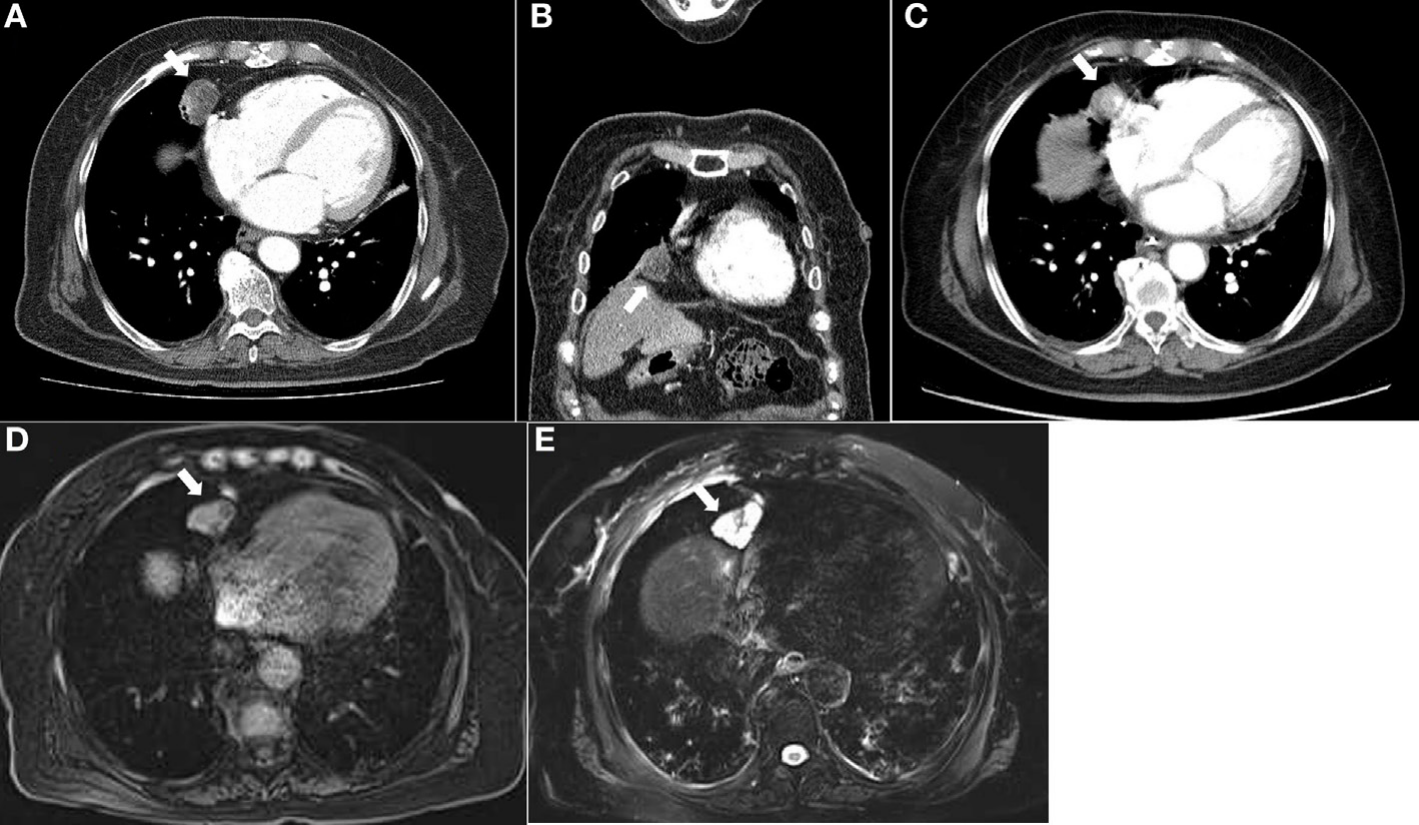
Computed tomography of the chest demonstrated a round-shaped, poorly enhancing mass with cystic portions measuring 2.7 cm at the right anterior cardiophrenic angle in the axial (A) and coronal (B) images. This lesion was observed on an incidenta abdominal CT scan taken 3 years ago. (C) Magnetic resonance imaging of the chest showed a lobulating mass at the right anterior cardiophrenic angle, with intermediate signal intensity on the T1-weighted image (D) and high signal intensity on the T2-weighted image (E).
